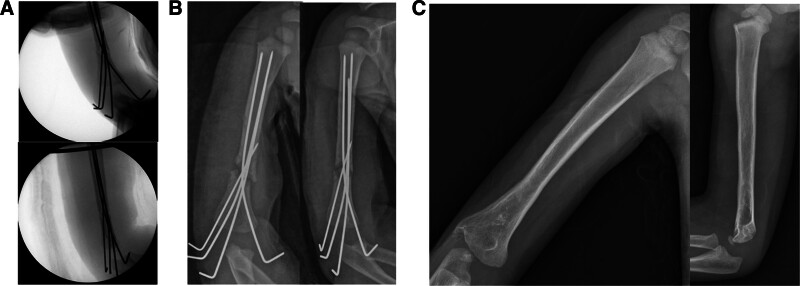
(A) Acceptable reduction was achieved via intramedullary nailing using Kirschner wires according to fluoroscopic images. (B) Postoperative radiographs of the second surgery. (C) Radiographs at 1 year and 6 months after the 2nd surgery.
